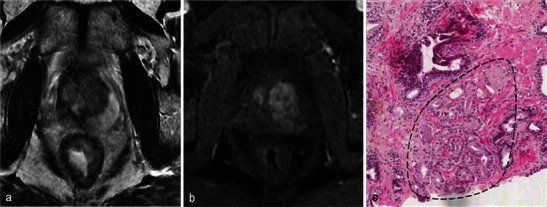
Small recurrence not seen on MRI. Patient with a positive right base prostate biopsy (3-mm focus). Six-month post-treatment axial T2-weighted (a) and axial dynamic contrast-enhanced (b) images show atrophy of the right lobe including the peripheral prostate; no suspicious nodules are visible. Pathology slide at high magnification (c) of a right base prostate biopsy, shows a small glandular focus (*circled*) between normal glands, lined by a single layer of cells
